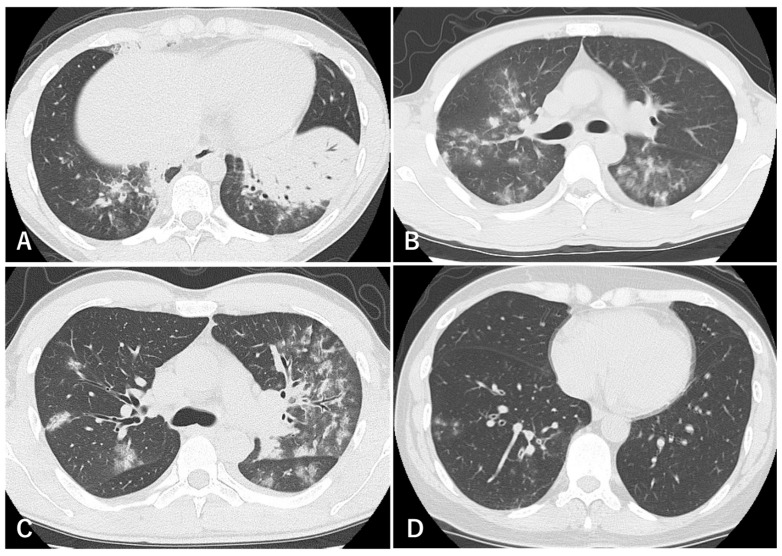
Representative thoracic computed tomography findings in adult *M. pneumoniae* pneumonia. Thoracic CT demonstrates dense consolidation in the left lower lobe with air bronchograms (A). Ground-glass opacities in a peribronchovascular distribution are shown in (B,C), accompanied by peribronchovascular thickening (C). Centrilobular nodules with bronchial wall thickening are shown in (C,D). GGO, ground-glass opacity. Reproduced from Ref. [44], with permission.
